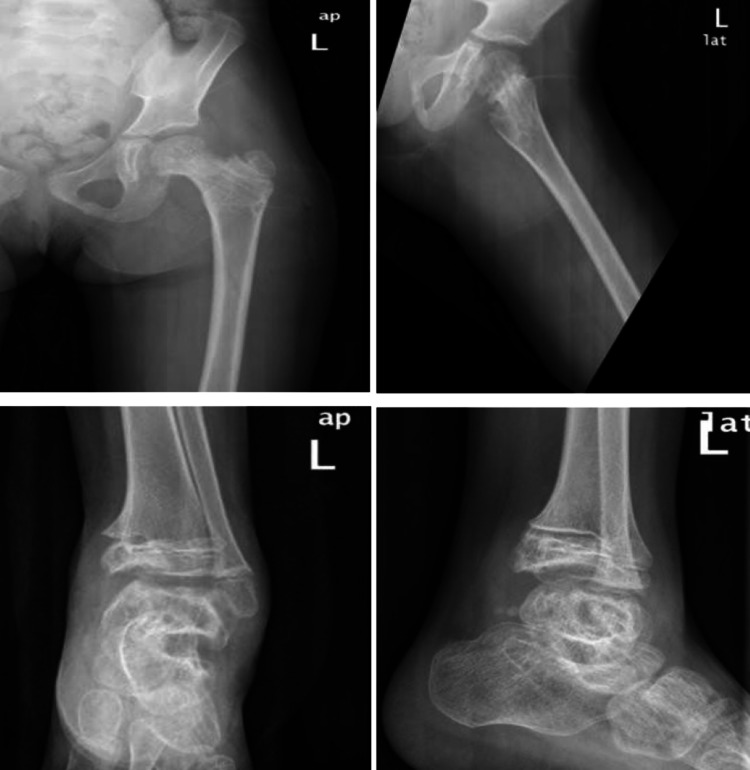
X-ray from a two-year follow-up with implant removal
